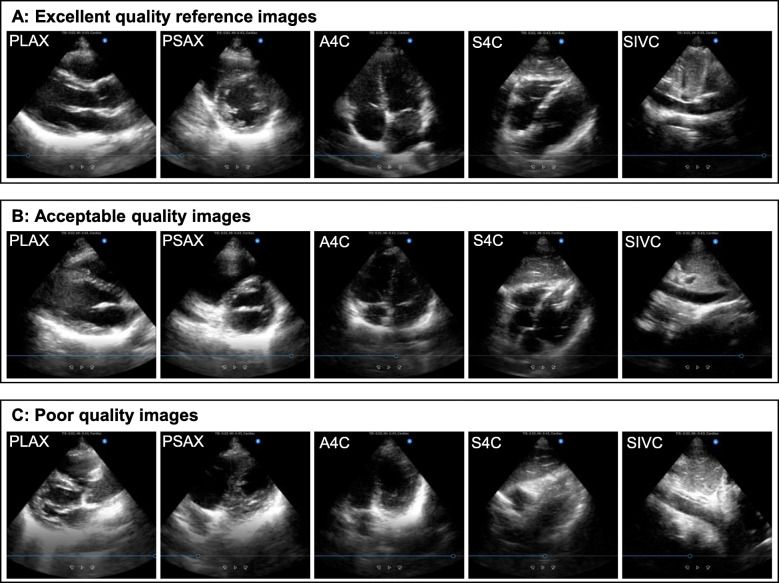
Excellent quality reference (A) and examples of acceptable (B) and poor quality (C) images of 5 cardiac POCUS views. Legend: Excellent quality reference images (A) refer to 5 cardiac POCUS views obtained by the cardiologist (MI) on the healthy volunteer (SJ) used for all skill tests. Examples of acceptable (B) and poor quality (C) images refer to the 5 views obtained by medical students on the volunteer. The excellent quality reference image (A) of the PLAX demonstrates all 7 structures (LA, LV, LVOT, RV, AV, MV, and IVS); the PSAX demonstrates all 4 structures (round LV, RV, papillary muscles, and IVS); the A4C demonstrates all 8 structures (LA, LV, RA, RV, MV, TV, IAS, and IVS); the S4C demonstrates all 7 structures (LA, LV, RA, RV, IAS, IVS, and liver); and the SIVC visualizes the IVC in a longitudinal fashion, the clear connection of IVC to RA, and the IVC diameter ≥ 1.0 cm at 2 cm from RA-IVC junction. The acceptable quality image (B) of the PLAX does not visualize AV; the PSAX is the mitral level of parasternal long-axis view, not the papillary muscle level; the A4C demonstrates a severely foreshortened LV; the S4C misses the LA and adds the aortic outflow (5-chamber view); and the SIVC does not visualize a clear connection of IVC to RA. The poor quality image (C) of the PLAX does not visualize the AV and the LV is severely foreshortened; the PSAX does not demonstrate a round LV and misses the papillary muscles; the A4C demonstrates a severely foreshortened LV and misses the LA; the S4C misses the RV and does not visualize the LA and the LV well; the SIVC demonstrates the descending aorta instead of the IVC. *POCUS*, point-of-care ultrasound; *PLAX*, parasternal long-axis view; *PSAX*, papillary muscle level of parasternal short-axis view; *A4C*, apical 4-chamber view; *S4C*, subcostal 4-chamber view; *SIVC*, subcostal inferior vena cava view; *LA*, left atrium; *LV*, left ventricle; *LVOT*, left ventricle outflow tract; *RV*, right ventricle; *AV*, aortic valve; *MV*, mitral valve; *IVS*, interventricular septum; *RA*, right atrium; *TV*, tricuspid valve; *IAS*, interatrial septum; *IVC*, inferior vena cava
